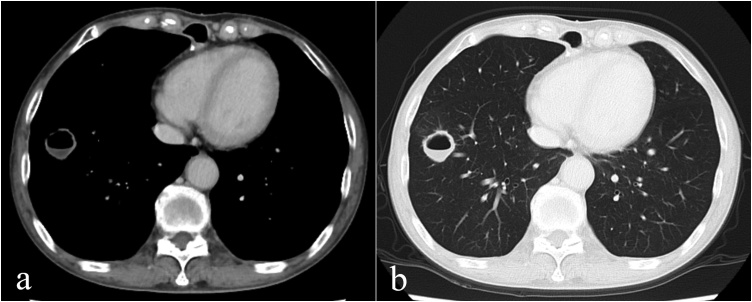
CT findings (18 months after surgery), a/b: mediastinal window/lung window. Cavitary lesion increased slightly in size, with wall thickening and fluid inside.
